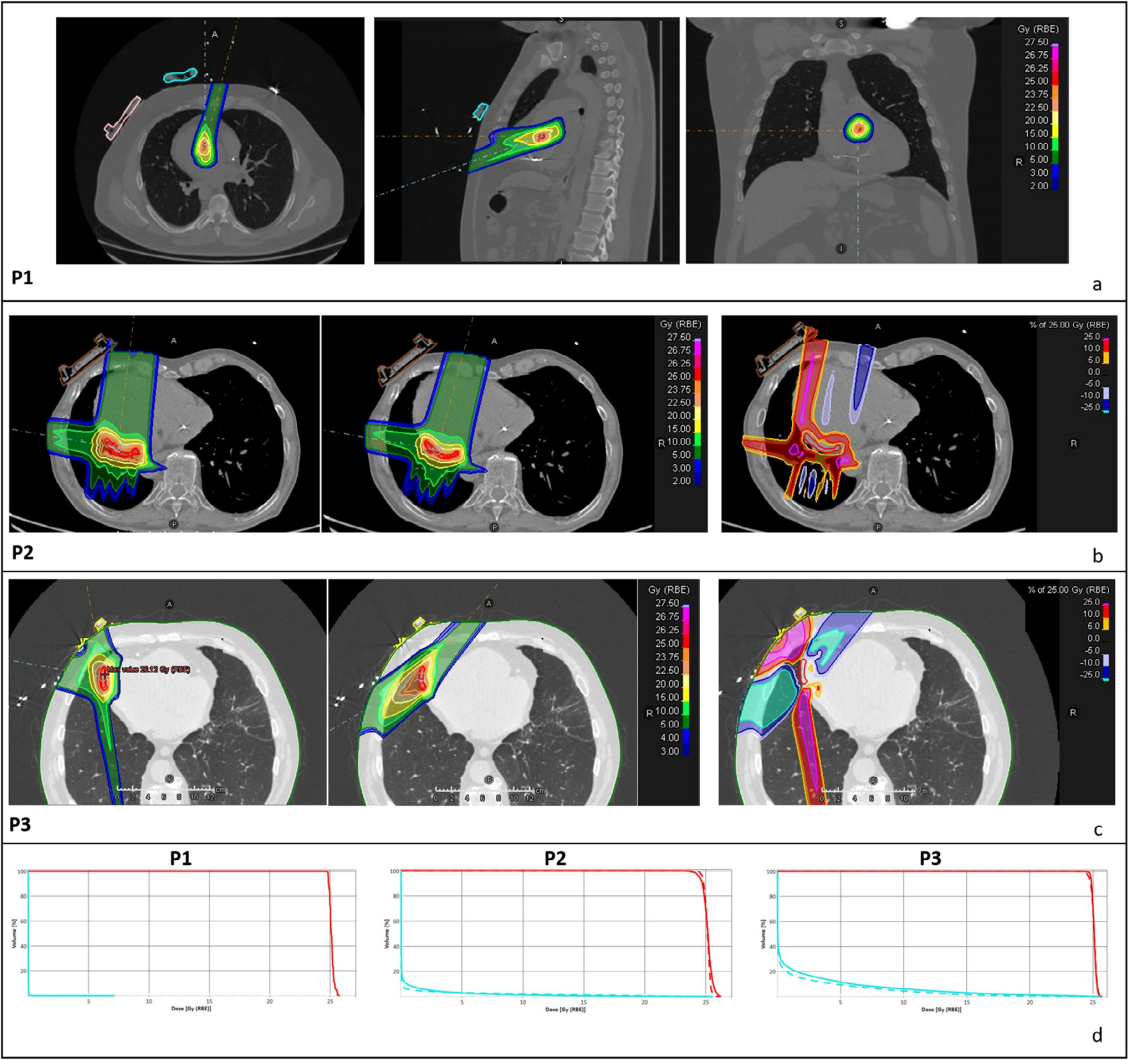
Representative treatment plans. (a) Best‐case scenario: example of a treatment plan that was not affected by the probe placement. The apical and parasternal probes are delineated by a pink and blue contour respectively and the beams’ geometry is represented by the orange and blue dashed lines for the transversal, sagittal and coronal view. (b) Mid‐range‐case scenario: example of a treatment plan with a slightly modified beam geometry to avoid intercepting the apical probe (brown contour). From left to right: dose distribution of the reference plan, dose distribution of the edited plan and dose difference between the two. The difference is expressed in percentage relative to the dose prescription. A negative difference means that the dose is higher compared to the reference plan. (c) Worst‐case scenario: this treatment plan demonstrates major modifications in beam geometry. To avoid the probe, a larger volume of the left lung receives medium‐high doses. Furthermore, a greater air gap exists between the beam entrance and the target compared to the reference plan, potentially reducing the treatment plan's robustness. (d) Dose volume histograms (DVHs) corresponding to the treatment plans shown above. The solid line represents the nominal plan, and the dashed line shows the modified plan. Red and blue colors denote the target and lungs statistics, respectively. Each DVH is labeled with a patient ID (P1, P2, P3) to match it with its corresponding dose distribution.
